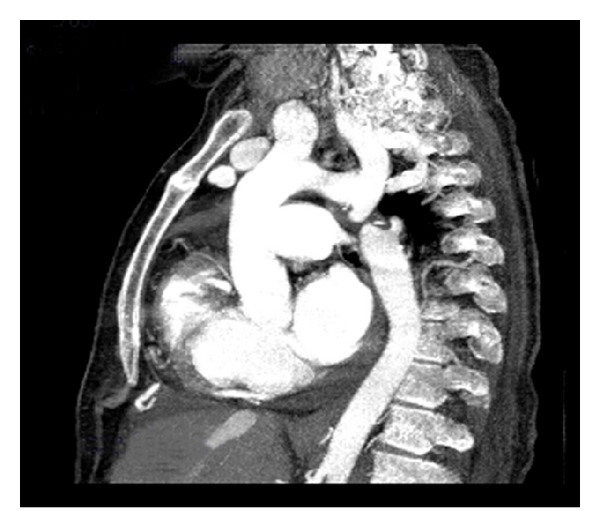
CT angiography showing type A interrupted aortic arch distal to the left subclavian artery.
